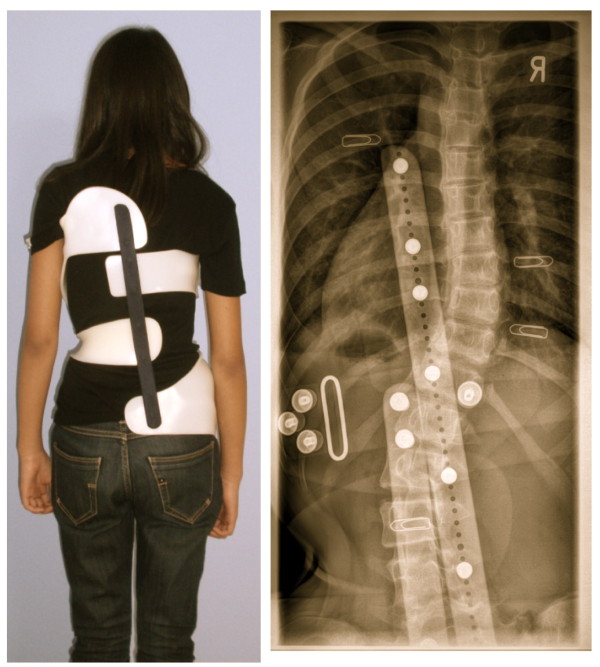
In-brace x-ray with pad markers visible. The markers are attached to the apical zones of the pressure areas. In this case an artificial decompensation, caused by the brace is clearly visible. In Double Major curvatures compression effects may arise in the middle of the trunk when the lower ribs are very long inhibiting thoracic realignment, when pushed by the lumbar pad. In this case, the brace has to be reassembled, because the correction effect is not sufficient. Without in-brace x-ray this imbalance would have remained undetected.
